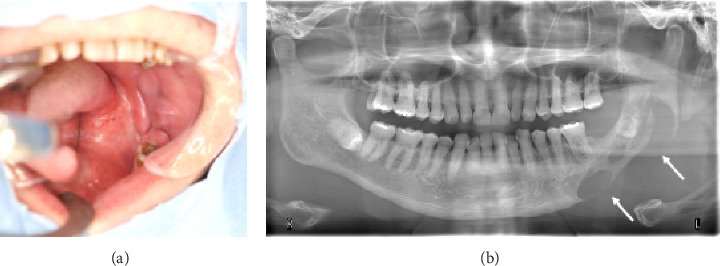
Oral findings at the time of examination. No abnormalities were observed in the mucous membrane (a). Panoramic radiograph showing destruction of the mandible. The white arrow indicates destruction of the mandible caused by the tumor (b).
